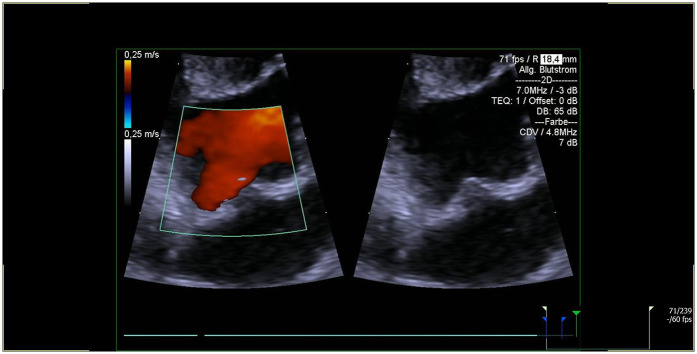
Transthoracic echocardiography, subcostal four-chamber view, of a thick interatrial septum with complete FO closure.
